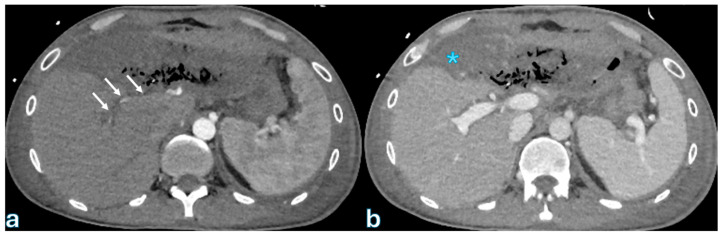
CT angiography of the abdominal vessels in (a) arterial and (b) portal venous phase showing nonopacification of the common hepatic artery (white arrows) and left portal branch, with subsequent ischemia of the corresponding liver parenchyma (blue asterisk). This finding was observed in a patient who underwent major pancreatic resection for pancreatic head adenocarcinoma four days after surgery and is consistent with embolization-induced liver infarction.
